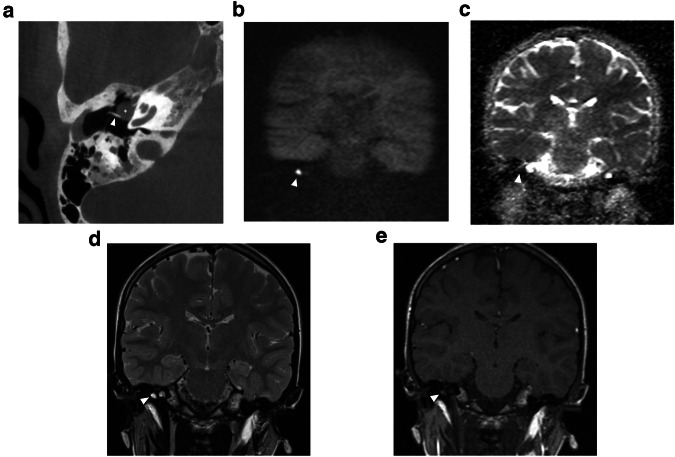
Eleven-year-old boy with conductive hearing loss in the right ear. a Axial CBCT image of a congenital middle ear cholesteatoma (CMEC) presenting as a well-rounded intratympanic mass in the anterior-superior quadrant (asterisk) and expanding medial to the malleus handle (arrowhead in a). b Non-EP DWI b1000, (c) calculated ADC map, (d) coronal TSE T2-weighted image, and (e) coronal TSE T1-weighted image: the white arrowhead points to the same lesion in these four images. The lesion has a high signal intensity on DWI and a low signal intensity on the ADC map. There is an intermediate signal intensity on T2-WI and a low signal intensity on T1-WI. The signal characteristics are almost pathognomonic of a cholesteatoma, in this case, a congenital cholesteatoma (CC)
